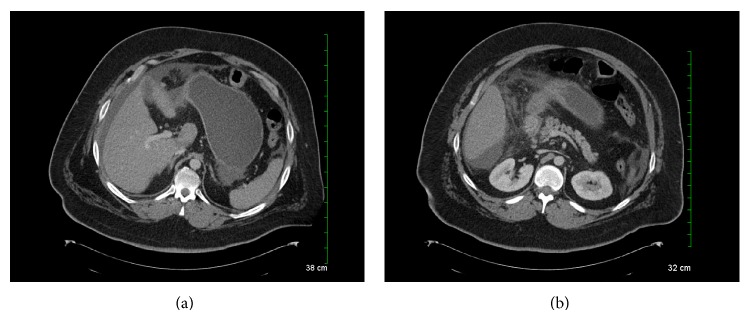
CT demonstrating a distended excluded stomach with perigastric and perihepatic ascites in the absence of pneumoperitoneum and an edematous duodenum with adjacent fat stranding.
